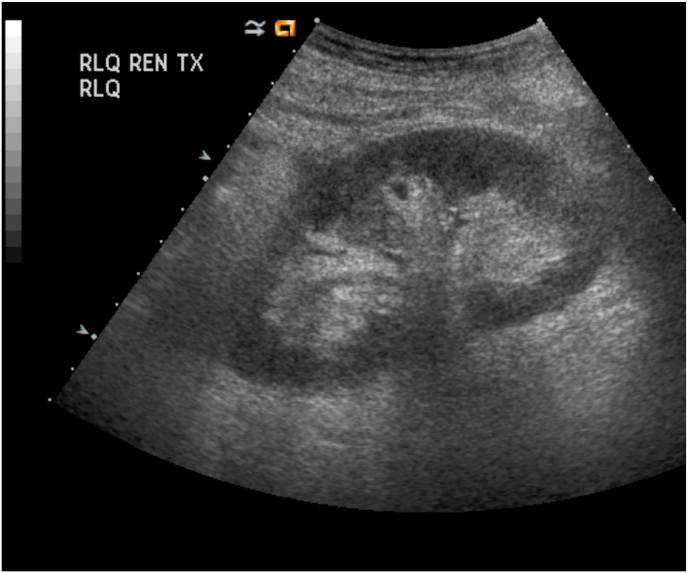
First post-transplant ultrasound showing normal graft kidney. (June 2014).
